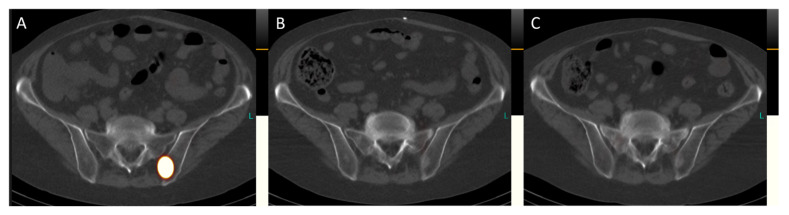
Transversal images of the post-therapeutic SPET/CT scans (Figure 6, (A), July 2023; (B), September 2023; (C), November 2023) reveal the complete remission of bone metastases. For instance, in the left ilium adjacent to the sacroiliac joint, a sclerosed lesion is now visible, indicating successful treatment.
